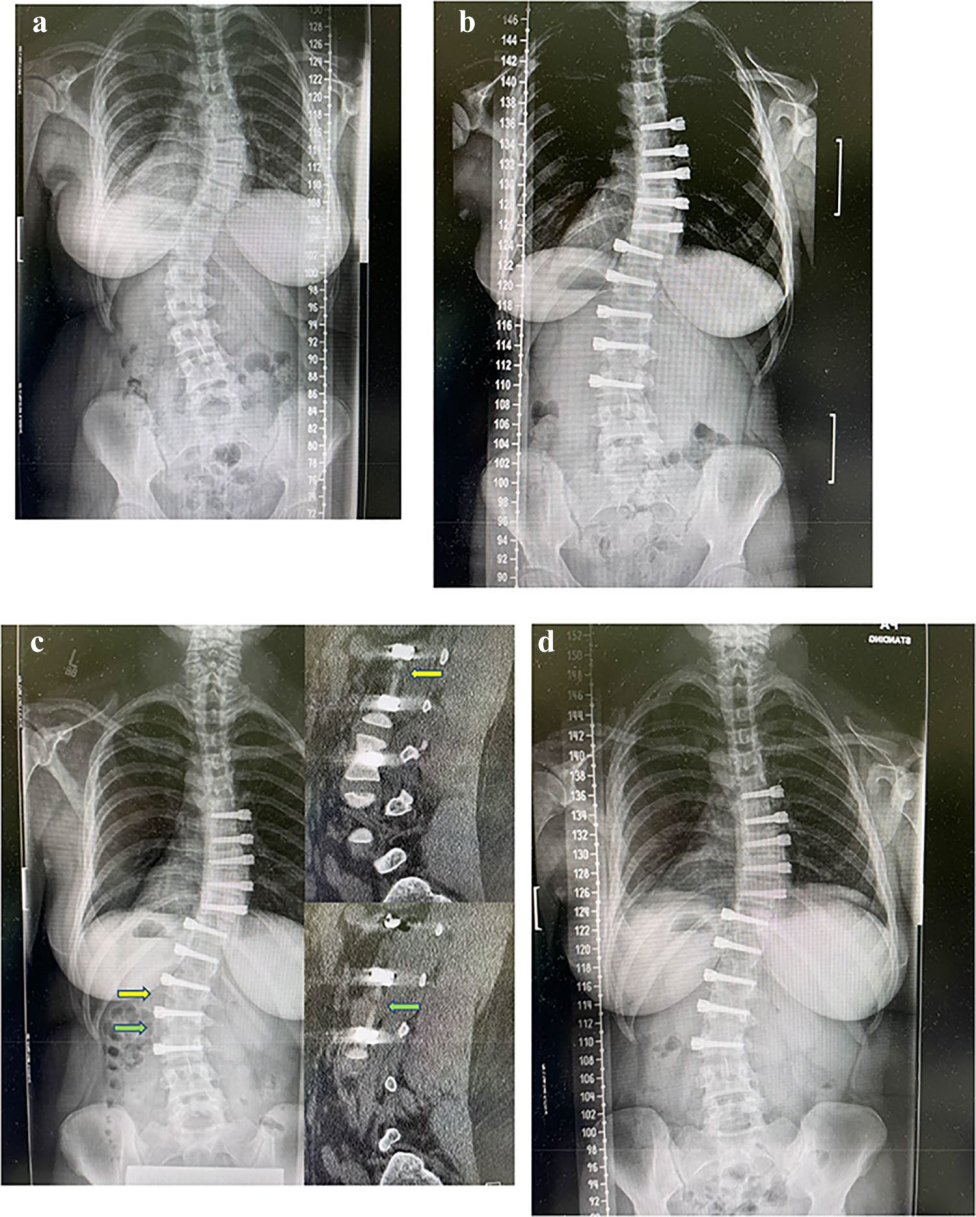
A–D (TIFF images 6–10). A 14 year old relatively skeletally mature female with progressive 47° right thoracic and 48° left thoracolumbar curves (Lenke 3C) at Risser 4 was treated with AVT right T6-10 and left T11-L3 with initial curve correction to 28° and 21°, respectively, with good control of both curves over 2 years. At 2 years, the thoracolumbar curve demonstrated 7° loss of correction to 28° with little change in the thoracic curve. Thoracolumbar tether rupture was suspected due to the change in angulation within screw pairs at L1,2 and L2,3. Mid-substance tether ruptures were confirmed at both levels on sagittal CT reconstructions. Despite a lack of pain or other symptoms, the patient and family were interested in revision AVT surgery. The tether was replaced uneventfully T11-L3 with curve correction to 24° and 16°, respectively. [A (TIFF image 7) Pre-operative posteroanterior radiograph; B (TIFF image 8) Postoperative posteroanterior radiograph; C (TIFF image 9) Follow-up posteroanterior radiograph at 2 years suggesting tether ruptures L1-3 and two CT sagittal reconstructions confirming tether ruptures at L1,2 and L2,3; D (TIFF image 10) Follow- up posteroanterior radiograph 1.5 years after tether revision]
